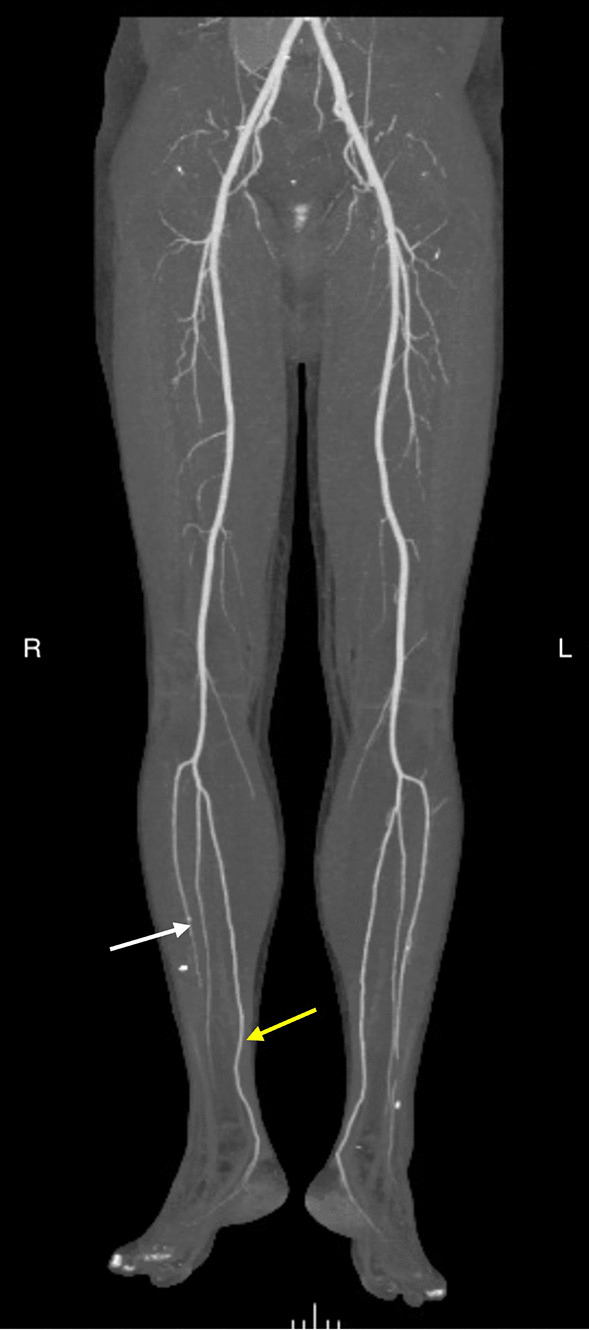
CT-angiography of the lower limbs demonstrating the right anterior tibial artery occluding in the distal leg (white arrow) with a patent right posterior tibial artery (yellow arrow). Note normal arteriogram of the left leg
